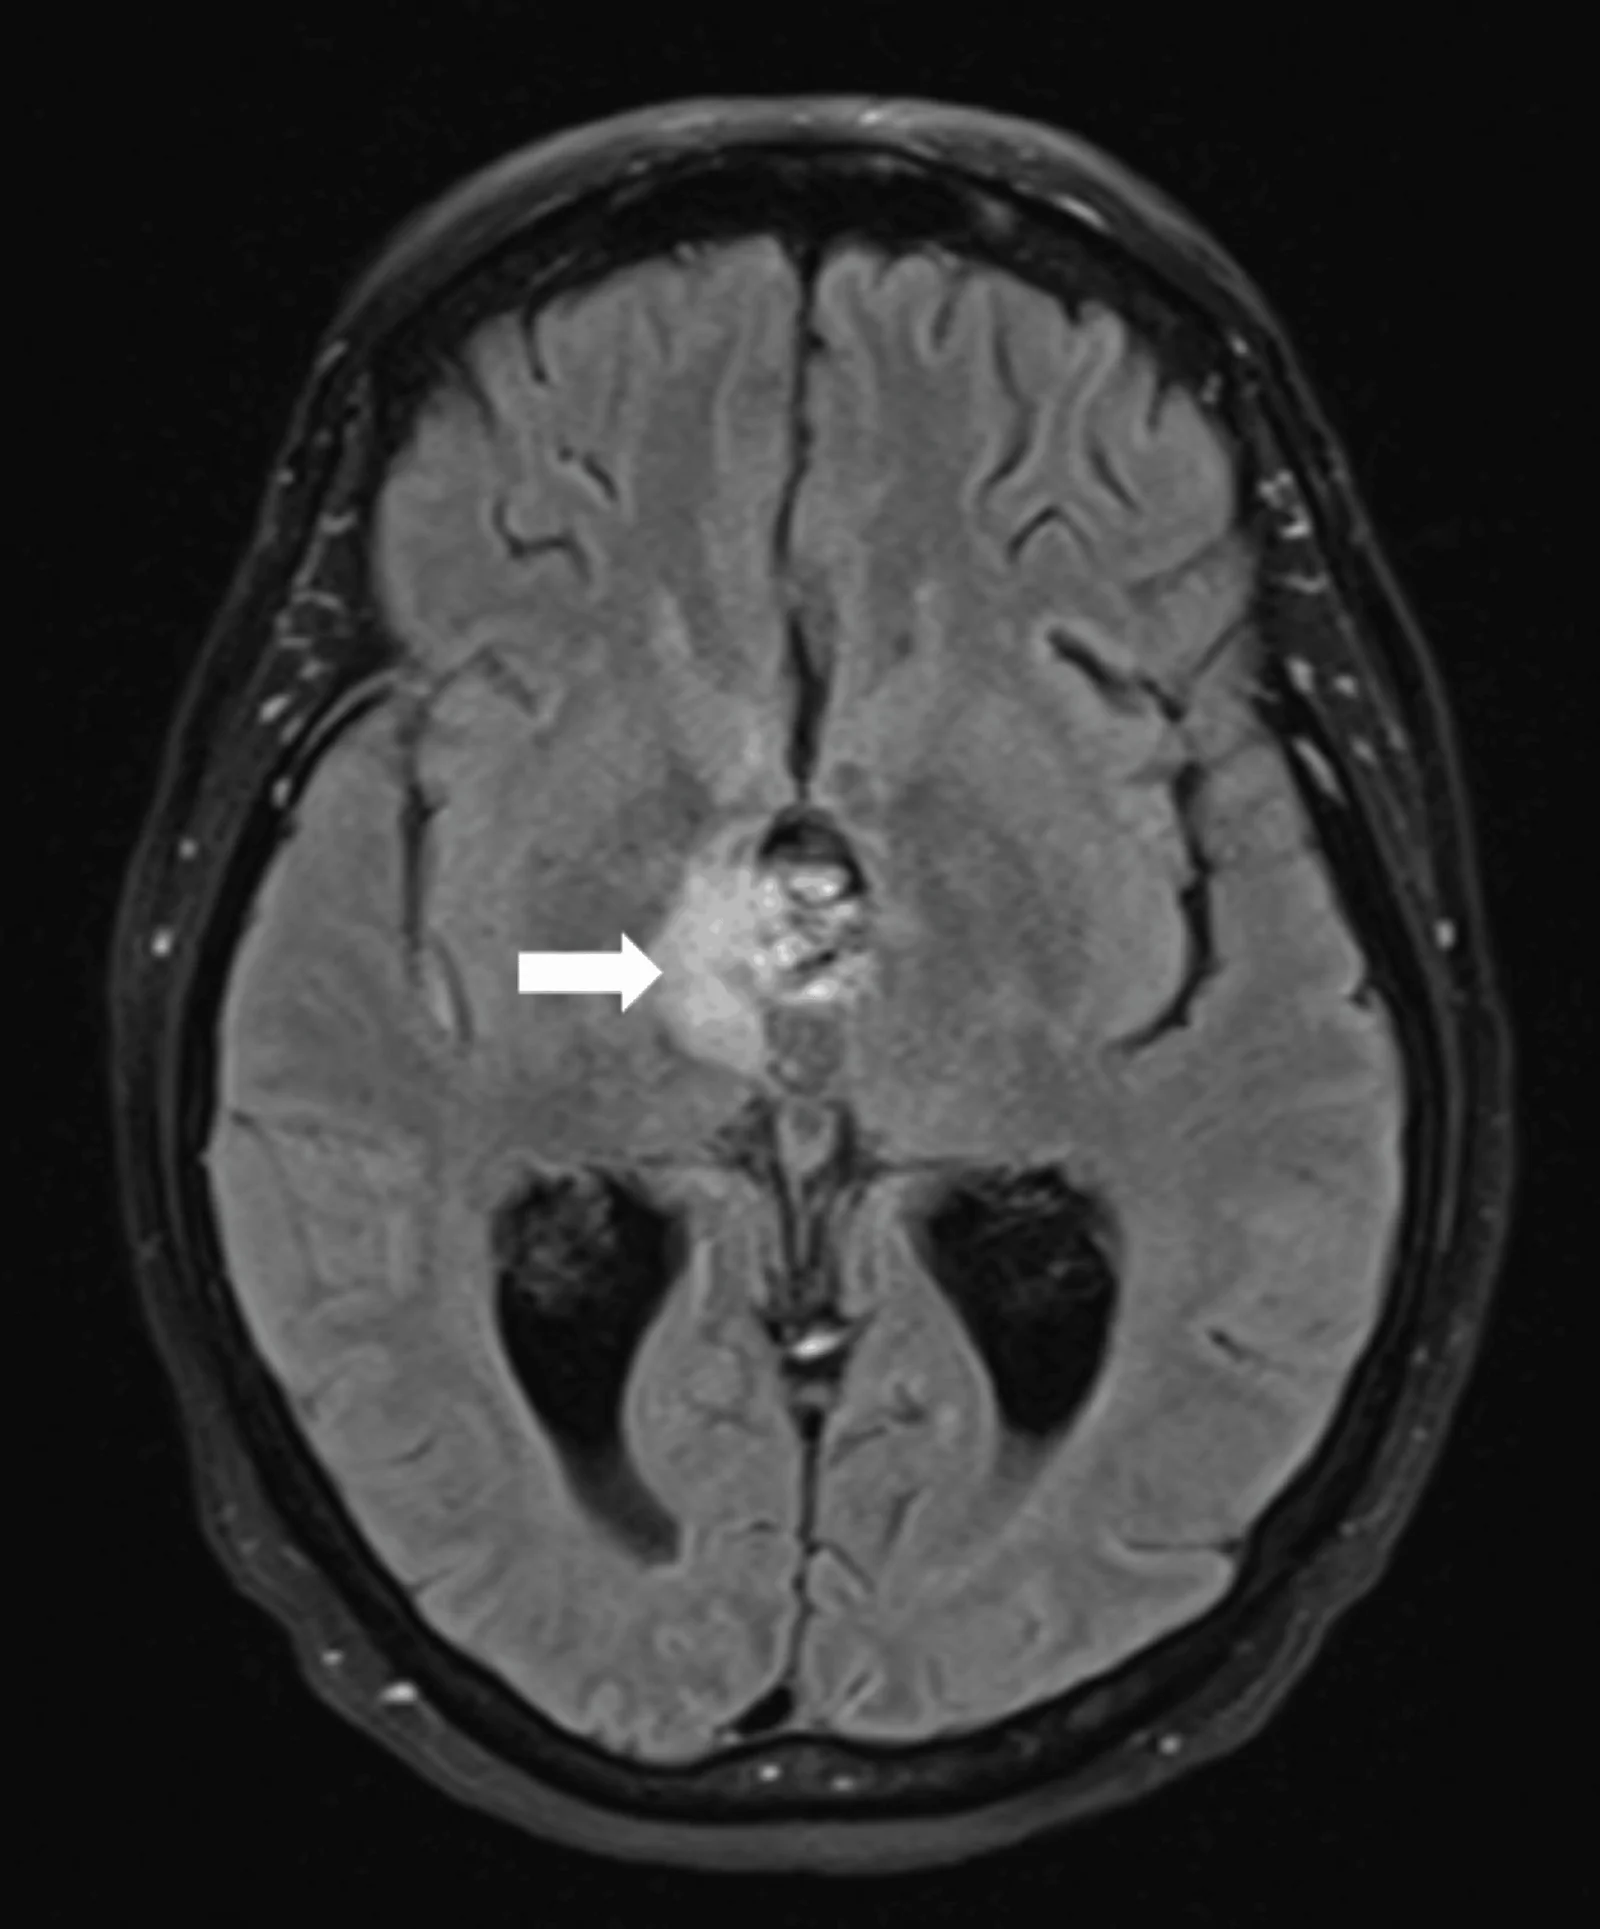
Post-embolization FLAIR (axial view) showing vasogenic edema and patchy enhancements in the right thalamus FLAIR: fluid-attenuated inversion recovery
